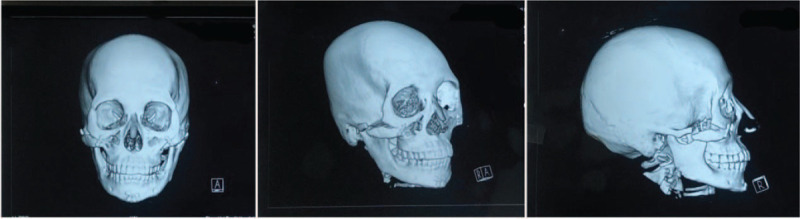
Preoperative computed tomography images of the patient showing bone malunion. The fractured root of the zygomatic arch was not fixed, and this resulted in downward movement of the bone mass and malunion of the bone mass in the right malar region.
